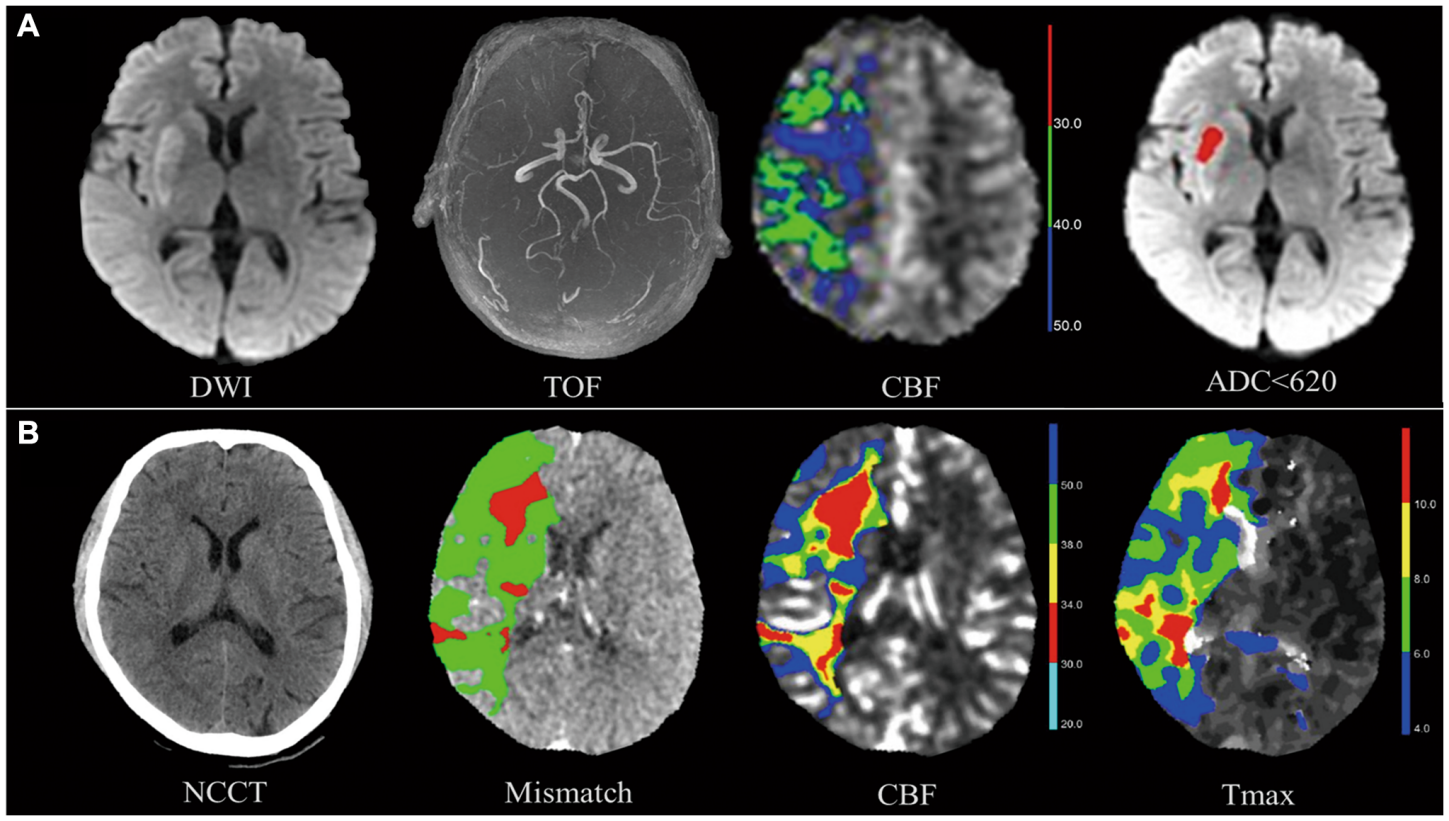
Hypoperfusion volume and ischemic core volume in arterial spin labeling (ASL) and computed tomography perfusion (CTP). The images above show two patients who suffered a right M1-MCA occlusion and neurological deficit (patient A admission NIHSS = 7, patient B admission NIHSS =12) and were successfully treated with thrombectomy (patient A mTICI 2C, patient B mTICI 3). (A) Top row shows patient A’s images. On TOF-MRA, DWI shows occlusion of right MCA with infarction of the basal ganglia. Ischemic core volume in ASL, measured by apparent diffusion coefficient (ADC), was 5.7 mL. The volume of ischemic core in ASL defined by apparent diffusion coefficient (ADC) < 620 was 5.7 mL. (B) Bottom row shows patient B’s images. The ischemic core volume in CTP defined by CBF < 30% (red) was 45.9 mL. A hypoperfusion volume of 285.1 mL was determined by the time to maximum (Tmax) >6 s (green). As defined by Tmax >6 s (green) - CBF < 30% (red), a mismatch volume of 239.2 mL has been observed. Both patients had excellent neurological recovery at discharge (NIHSS = 1).
